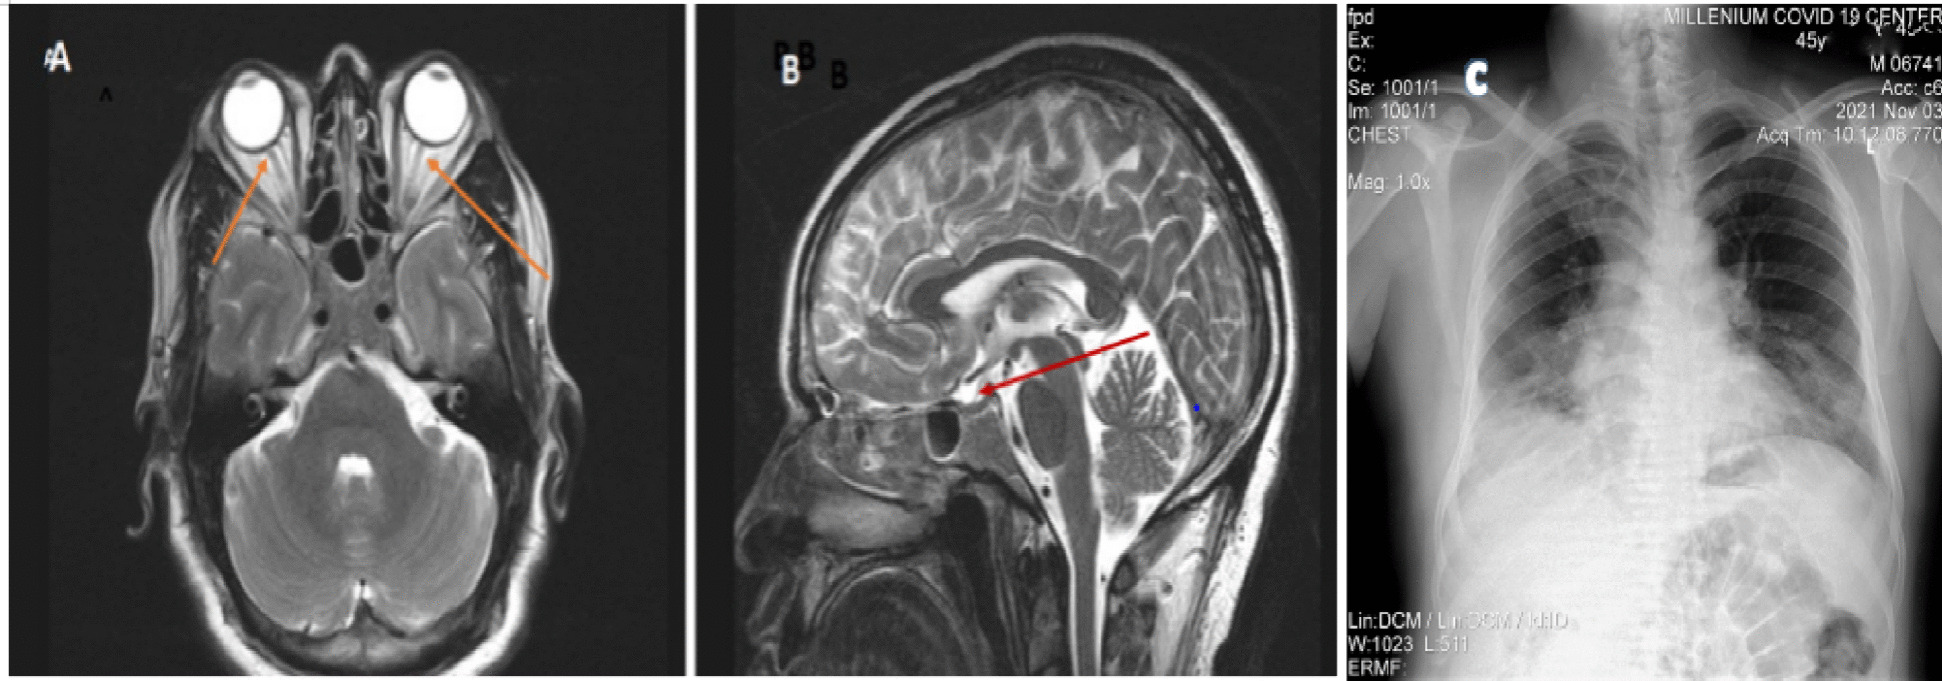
T2 axial and sagittal brain MRI scan showed prominent subarachnoid space around the optic nerves (A) and flattening of pituitary gland (B). Chest X-ray showed bilateral ground glass opacities mainly in the middle and lower lung zones with right side blunted costophrenic angle (C)
